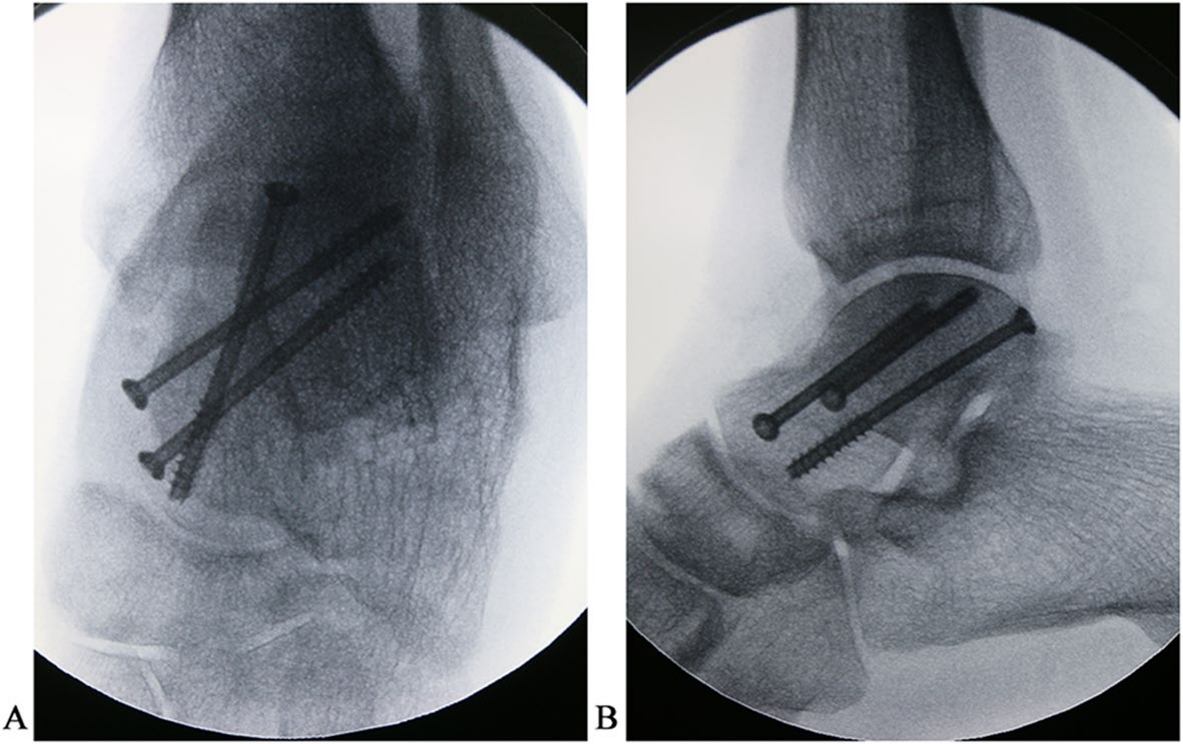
Immediate postoperative anteroposterior (A) and lateral (B) X-ray radiographs of the talar body fractures treated with arthroscopically assisted percutaneous osteosynthesis
